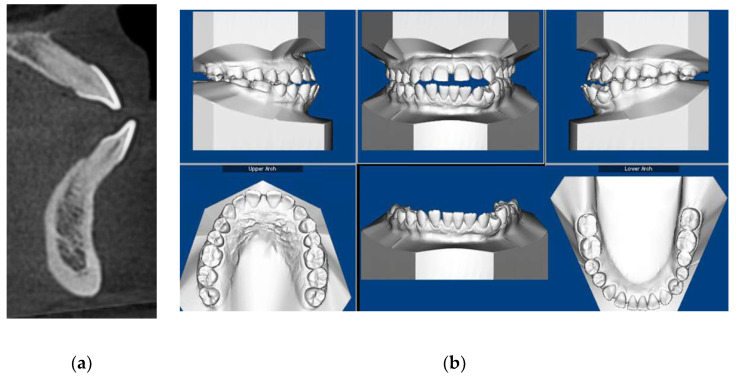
(a) Cone-beam computed tomographs (CBCT)-driven sagittal screen of lower incisor showing its severe proclination with no bone appears on most of the labial surface of the root; (b) Initial digital models.
